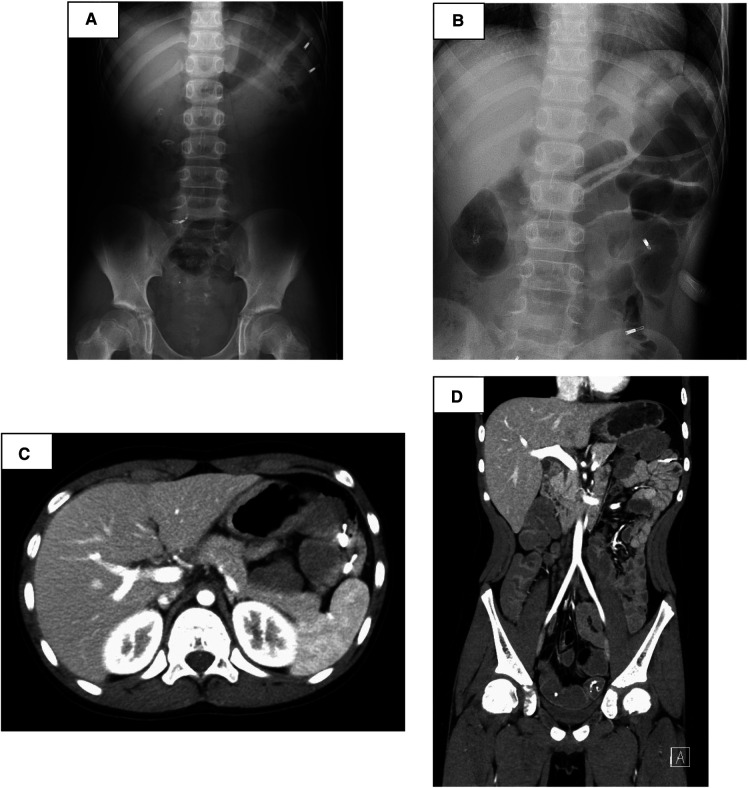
After endoscopic mucosal resection—suspected post-bleeding, abdominal x-rays (A) and enhanced abdominal computed tomography scan revealed that the position of the clips had not changed from the position immediately after EMR (B), and there were no signs of gastrointestinal perforation or arterial bleeding (C,D).
